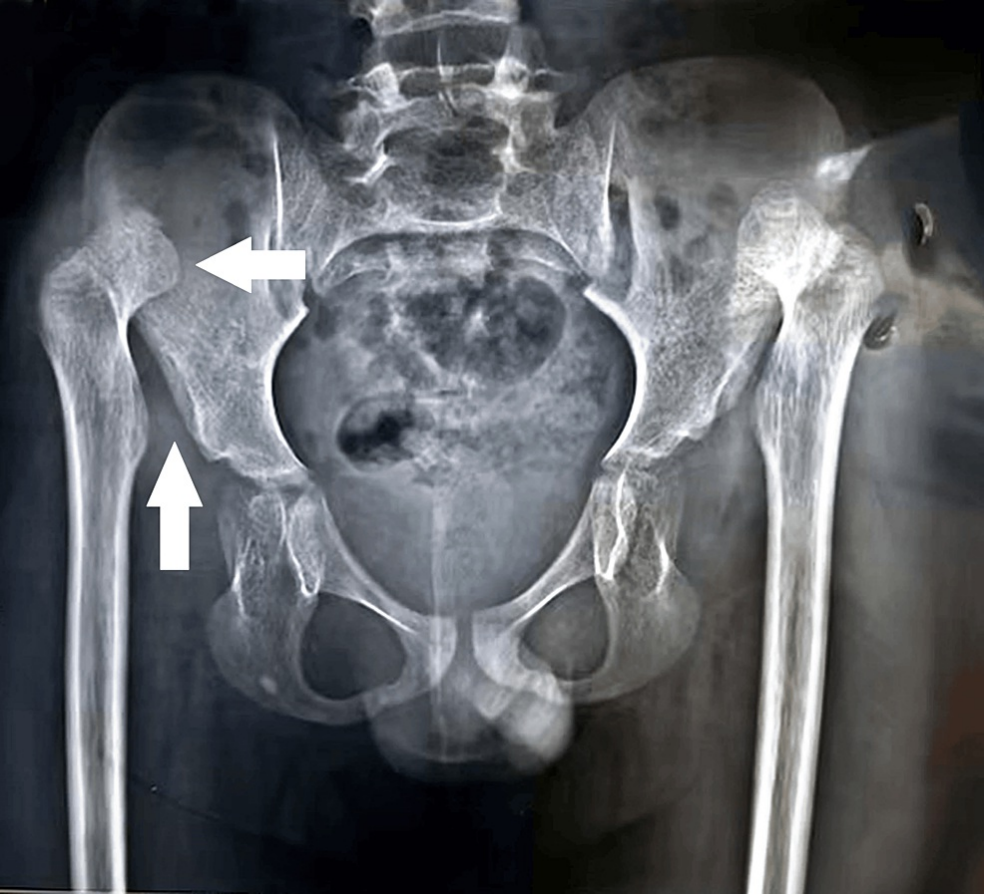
X-ray pelvis shows congenital hip dysplasia
